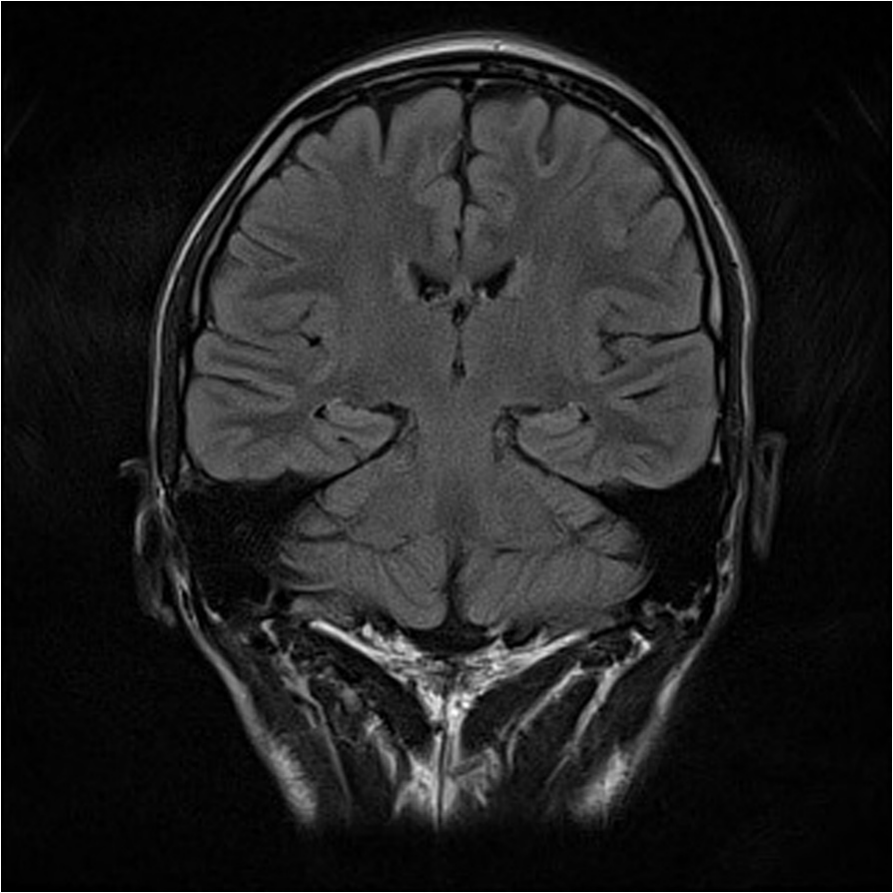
Coronal brain MRI with FLAIR sequence.
